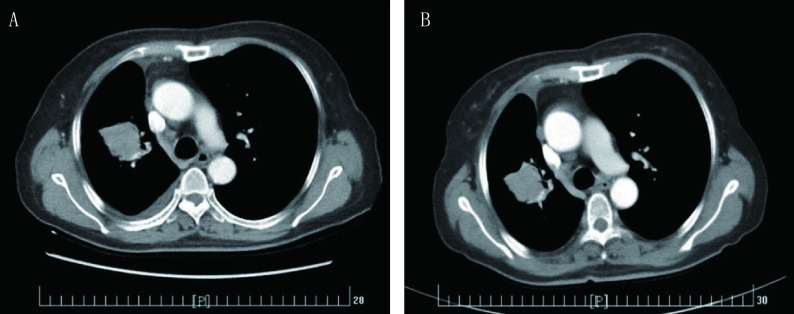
第二次培美曲塞应用。A：第二次应用前基线检查（2017年2月7日）；B：第二次应用最佳疗效（2017年3月28日） The second pemetrexed challenge. A: The baseline CT image before the second pemetrexed challenge (Feb. 7, 2017); B: The best response CT image after the second pemetrexed challenge (Mar. 28, 2017)
